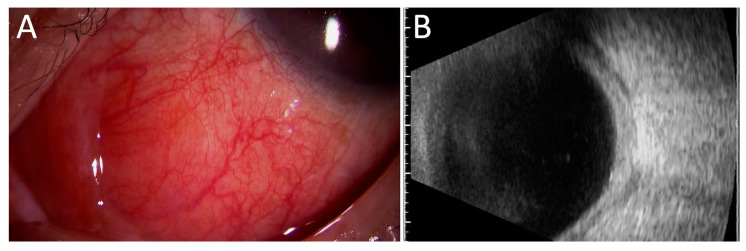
Nodular anterior and posterior scleritis associated with RPC: slit-lamp exam showed anterior nodular scleritis (A) and B-scan ultrasonogram (B) showed thickened sclera with fluid in the sub-Tenon’s space (T-sign).
